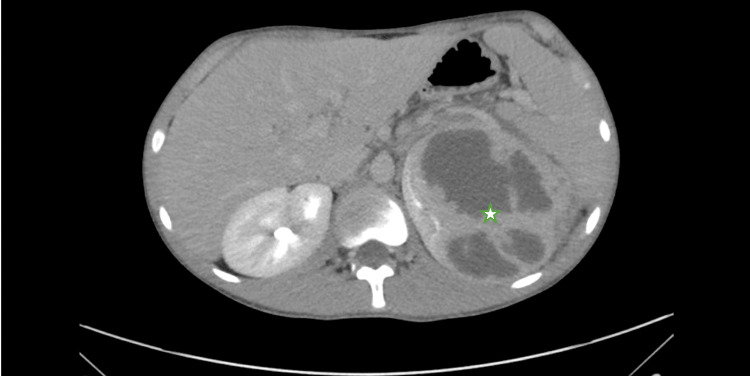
CT imaging (axial view) Demonstrating a complex solid and cystic mass involving the upper to mid pole of the left kidney measuring 11.5 x 7.9 x 10 cm (star), with multiloculation, septations, perinephric micro-collections, extensive perinephric stranding and fluid, and enhancing urothelial thickening of the upper left renal collecting system.
